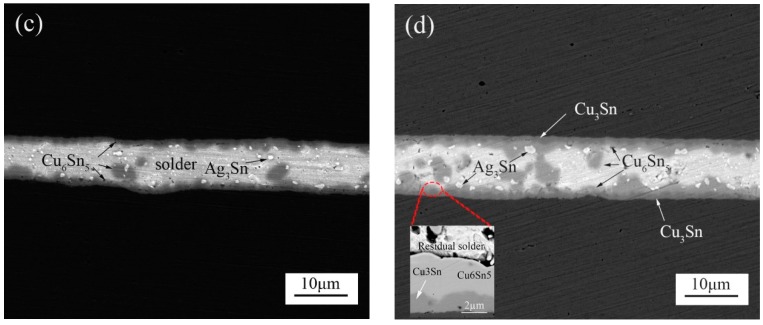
The morphologies of the cross-sectional Cu/SAC305/Cu joints formed at an 800-ms ultrasonic bonding time at different aging times of (a) 0 h; (b) 120 h; (c) 240 h and (d) 360 h. The inset in (d): the local enlargement image at the interfaces.
